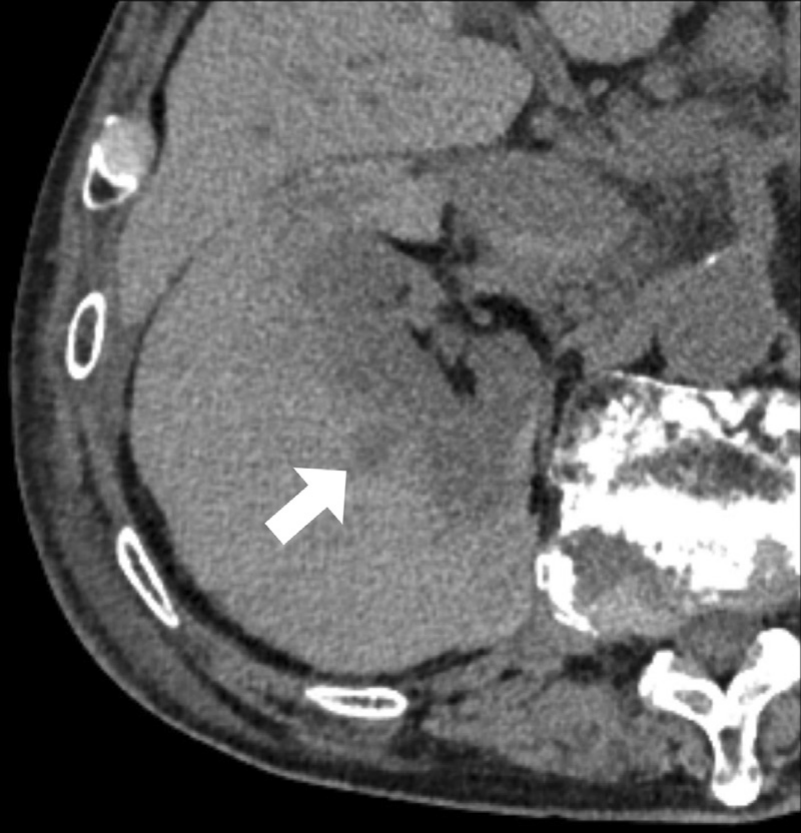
Non-enhanced PCD-CT shows a nodular low-density area (arrow) within the high-density area corresponding to the subcapsular hematoma surrounding the right kidney.
